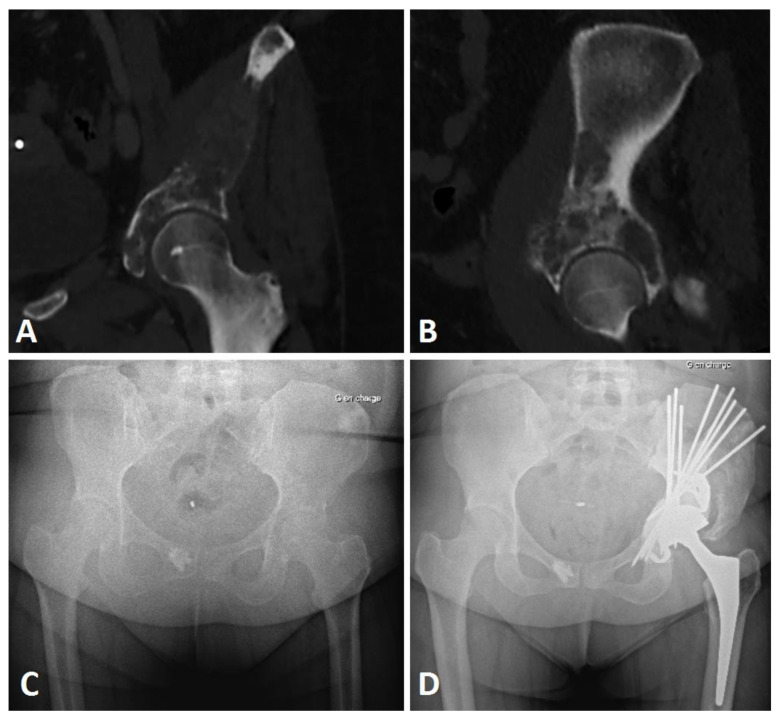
Imaging views of a breast metastatic iliac lesion Harrington class III. CT scan frontal (A) and sagittal views (B), preoperative AP X-ray (C), and postoperative (D) views of a Harrington procedure (Steinman pins and cement) associated with THA with acetabular reinforcement using a Kerboul cross-plate, in a metastatic localization of breast cancer with pelvic discontinuity at 2-year follow-up, Parker 9.
